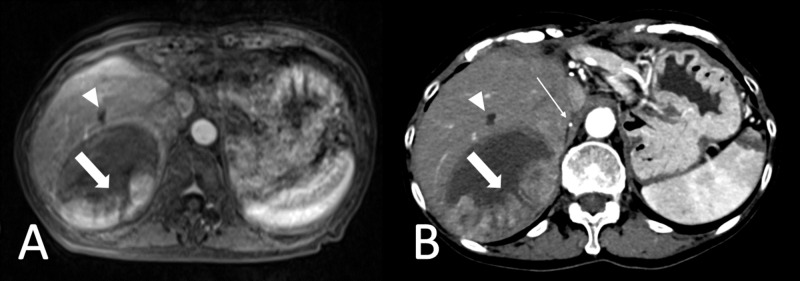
A) Axial MR image, T1-weighted 3D gradient-echo, delayed arterial phase. Status post TACE with a large embolization cavity and persistent peripheral enhancing nodular components (arrow). Partially visualized anterior to the embolization cavity is a simple cyst. B) Axial CTA image. Status post TACE with large embolization cavity with persistent peripheral enhancing nodular components (thick arrow) and adjacent hypertrophied right phrenic artery (thin arrow). Partially visualized hypodensity anterior to the lesion is a simple cyst. MR: magnetic resonance; 3D: three-dimensional; TACE: transarterial chemoembolization; CTA: computed tomographic angiogram
